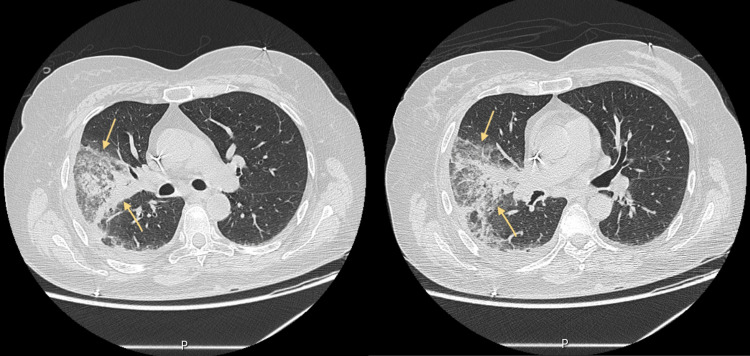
Chest computed tomography performed during ICU stay.
